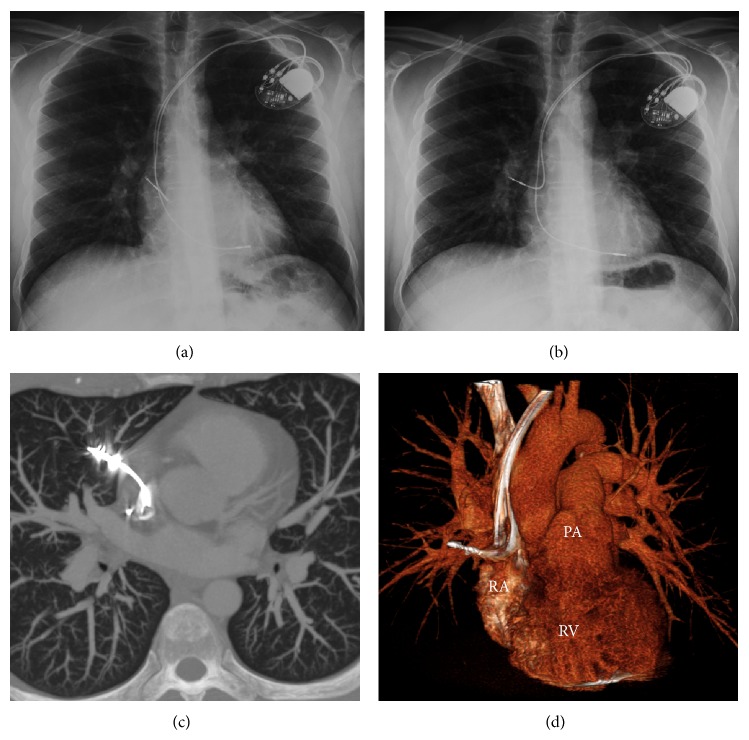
(a) Postimplant anteroposterior chest radiography showing the atrial lead positioned at the basal part of the right atrial appendage. (b) Anteroposterior chest radiography performed at 8 months showing a clear late migration of the atrial lead into the right lung. (c) Contrast-enhanced CT-scanner with maximal intensity projection (MIP) and (d) volume rendered reformatting (anterior view) demonstrating the migration of the atrial lead into the right lung. Notably the pericardium, pleura, and pulmonary parenchyma were normal. RA: right atrium; RV: right ventricle; PA: pulmonary artery.
